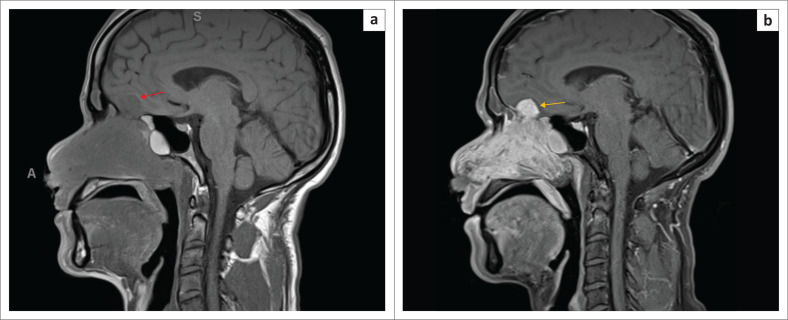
(a) T1 turbo spin echo (TSE) sagittal MR image shows a homogeneously mass, iso-intense to grey matter and muscle (red arrow). (b) Sagittal T1 turbo spin echo (TSE) with fat saturation (FS) with gadolinium-enhanced MR image shows a diffusely heterogeneous enhancing tumour in the left nasal cavity that transgresses the cribriform plate extending intracranially (orange arrow).
